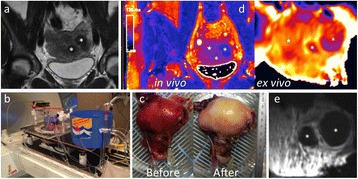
a In vivo MRI of uterus with hypointense T2wSI fibroids (asterisks). Ex vivo perfusion model. c Photos of Fig. 199. the excised uterus before and after perfusion. (d) MR T2 maps: fibroids are hypointense relative to myometrium (star). (e) Ex vivo TWIST MRI
